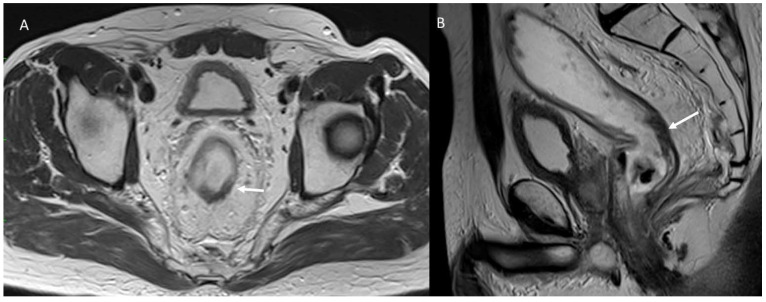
MRI assessment post n-CRT treatment: fibrotic response in T2-W axial (arrow) (A) and sagittal plane (arrow) (B).
